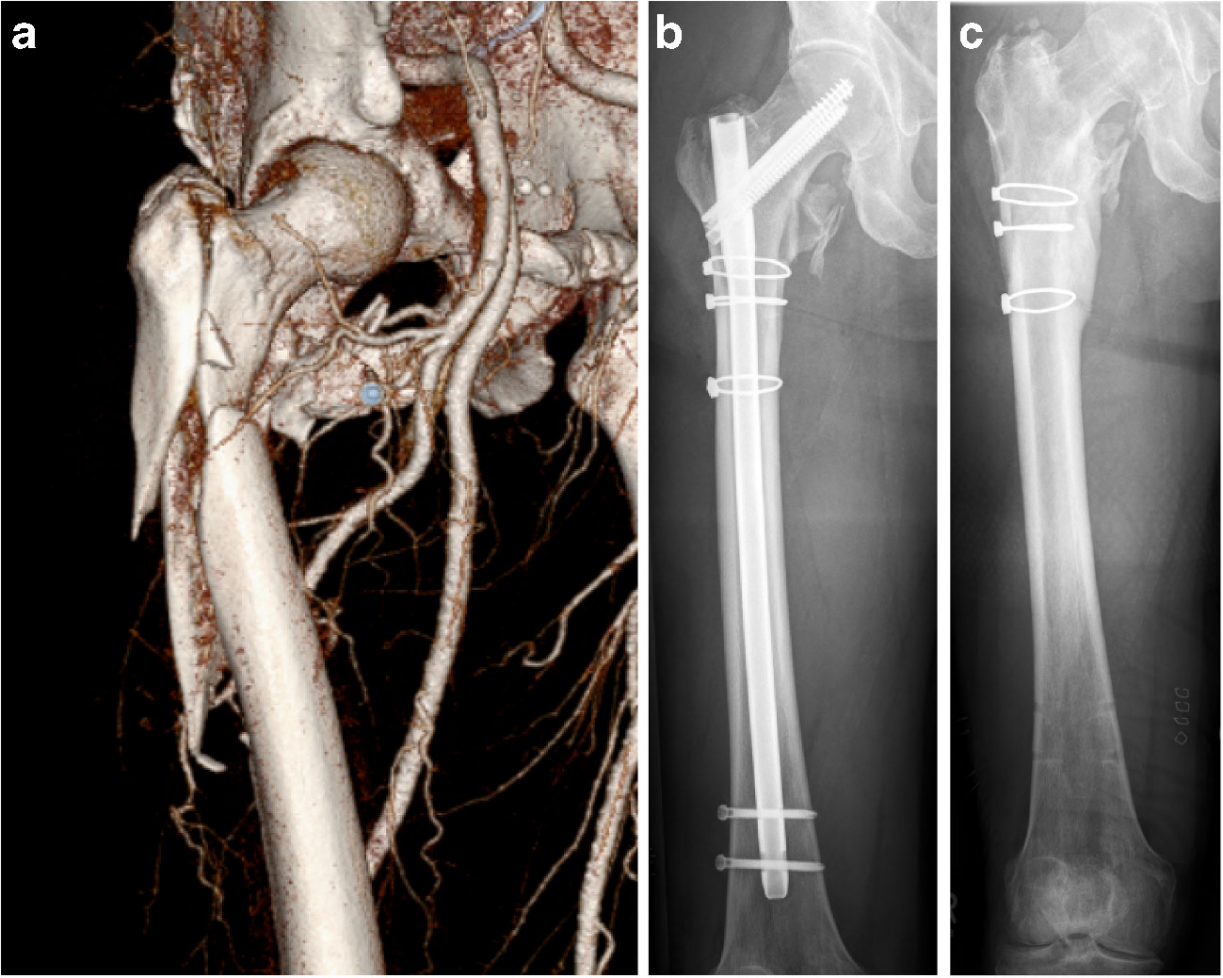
a CT scan demonstrating a subtrochanteric AO/OTA A3.3 fracture on the right side in a 62-year-old patient after motorcycle accident. b ORIF was performed using a reamed cephalomedullary nail and three auxiliary cerclages with the patient in the lateral decubitus position without any traction device and with free draping of the injured leg. c Follow-up 1 year after trauma demonstrating the situation after elective implant removal. The cerclages were left to avoid unnecessary tissue damage
